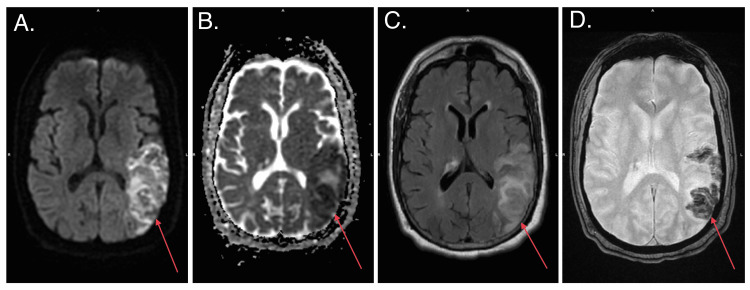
Brain MRI showing a subacute infarct in the left parietal lobe (red arrows).
